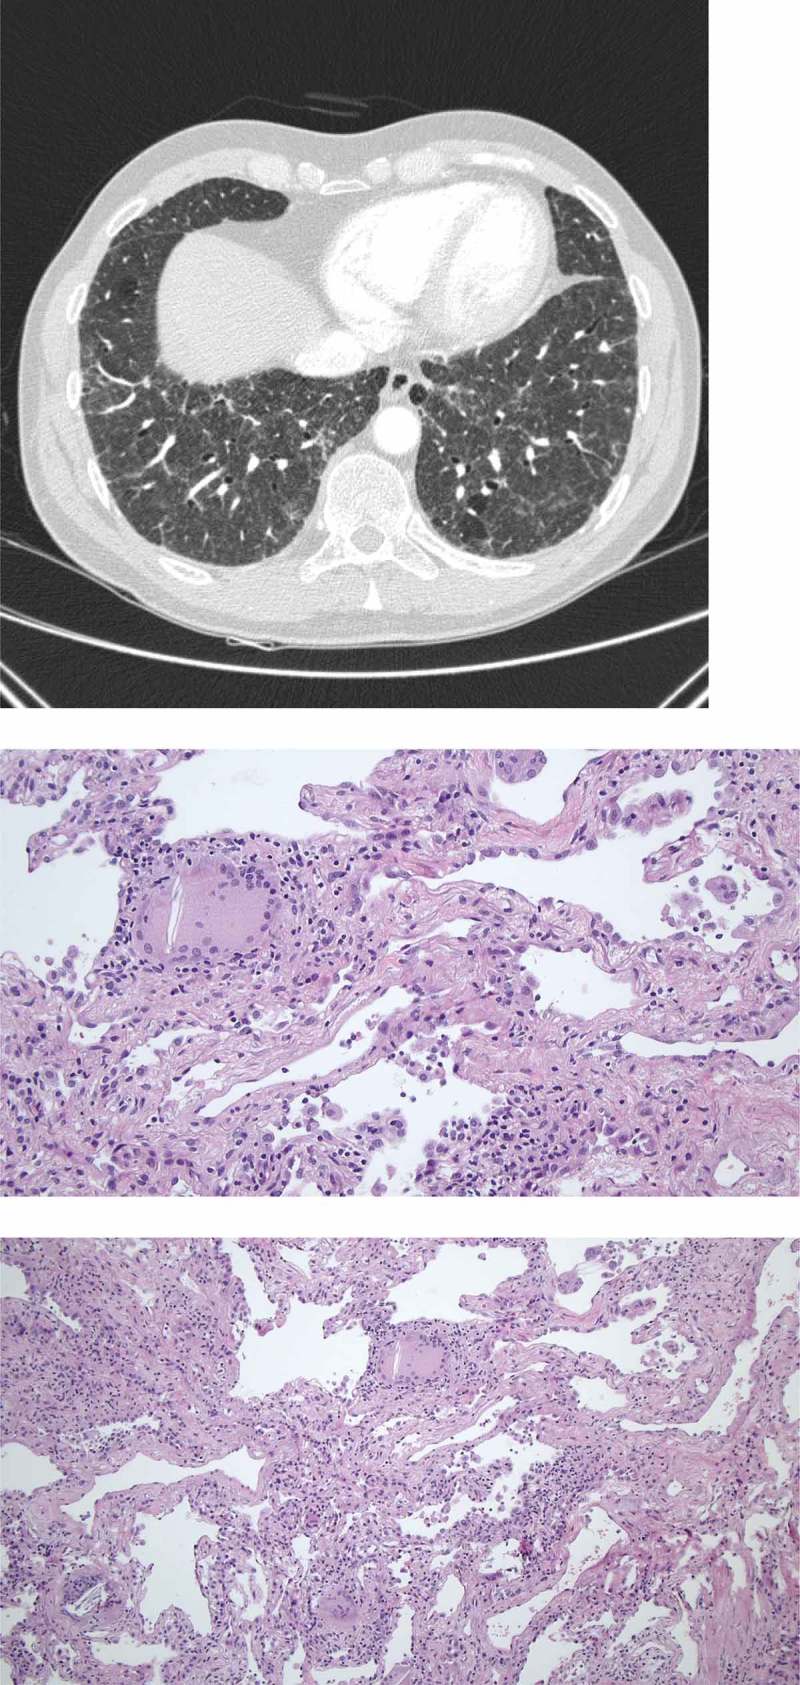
51-year-old male referred for increasing dyspnea and cough. HRCT showing diffuse reticulation, ground glass opacity, traction bronchiectasies and consolidations. Cryobiopsies showing chronic inflammation, fibrosis and granulomas/giant cells. The patient was diagnosed with chronic hypersensitivity pneumonitis.
